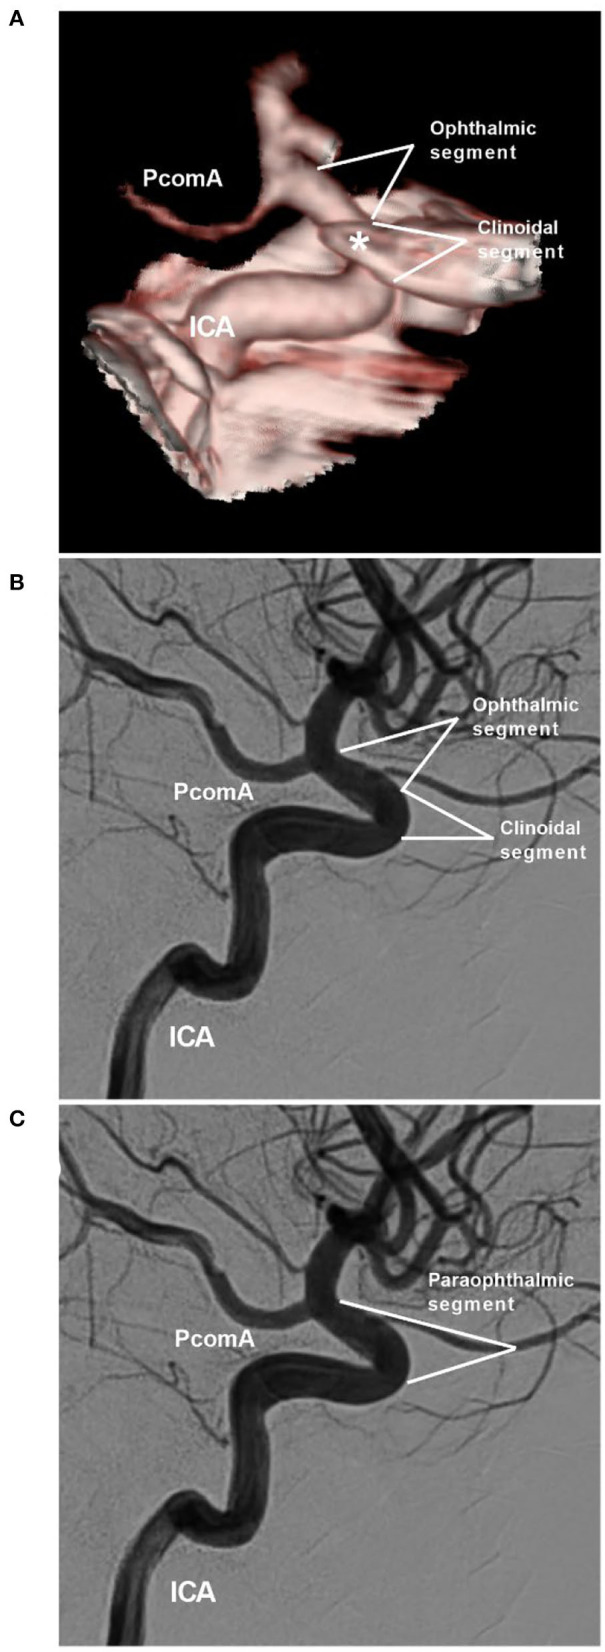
NYU paraophthalmic segment. (A,B) CTA (A) and DSA of the ICA (B) show the clinoidal and ophthalmic segments of the ICA. The segmentation is according to the classifications by Bouthillier and Ziyal. The asterisk in image (A) indicates the anterior clinoid process. (C) DSA shows the NYU segmentation. The clinoidal and ophthalmic segments of the ICA are combined into the paraophthalmic segment. CTA, computed tomography angiography; DSA, digital subtraction angiography; ICA, internal carotid artery; NYU, New York University; PcomA, posterior communicating artery.
